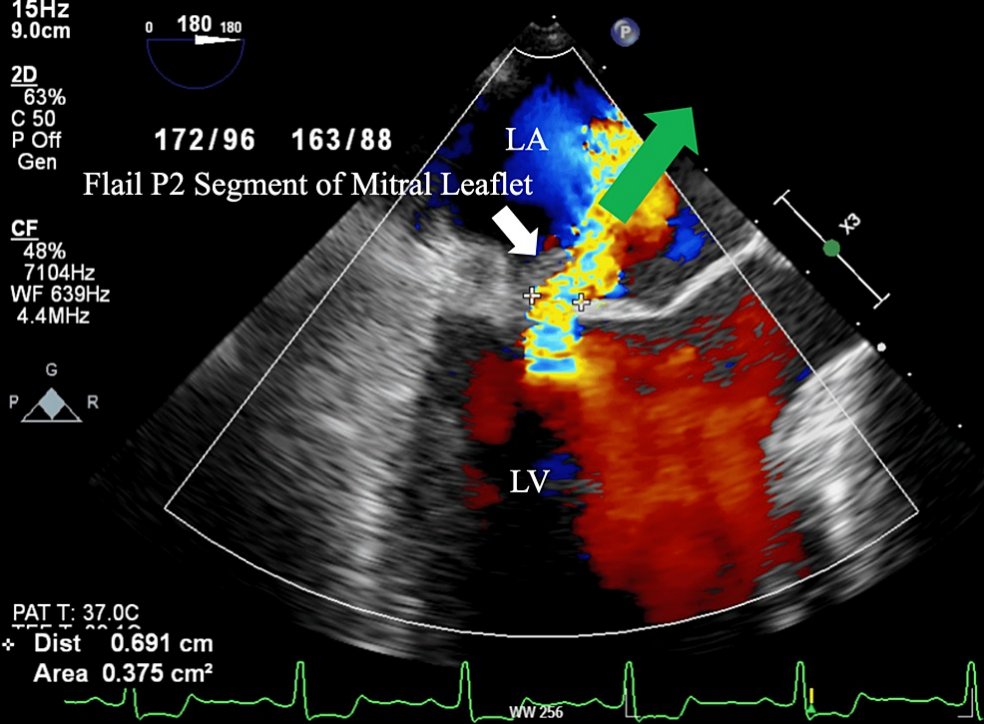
M-mode, mid-esophageal view of transesophageal echocardiogram showing flail mitral leaflet (white arrow) resulting in eccentric regurgitant flow (green arrow) from LV to LA. LA: Left atrium, LV: Left ventricle
